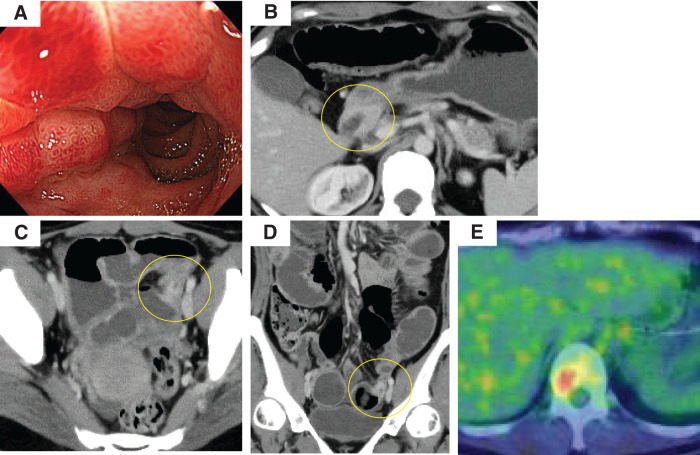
Imaging and endoscopic findings. (A) Upper gastrointestinal endoscopy showing mucosal erythema and edema with luminal narrowing in the duodenal bulb. (B) Contrast-enhanced CT demonstrating diffuse wall thickening with enhancement in the duodenum (yellow circle), corresponding to the endoscopic findings. (C, D) Axial (C) and coronal (D) contrast-enhanced CT images showing segmental wall thickening of the pelvic small intestine with luminal narrowing, leading to bowel obstruction (yellow circle). (E) PET-CT revealed abnormal uptake in the vertebral body, suggestive of bone metastasis.
